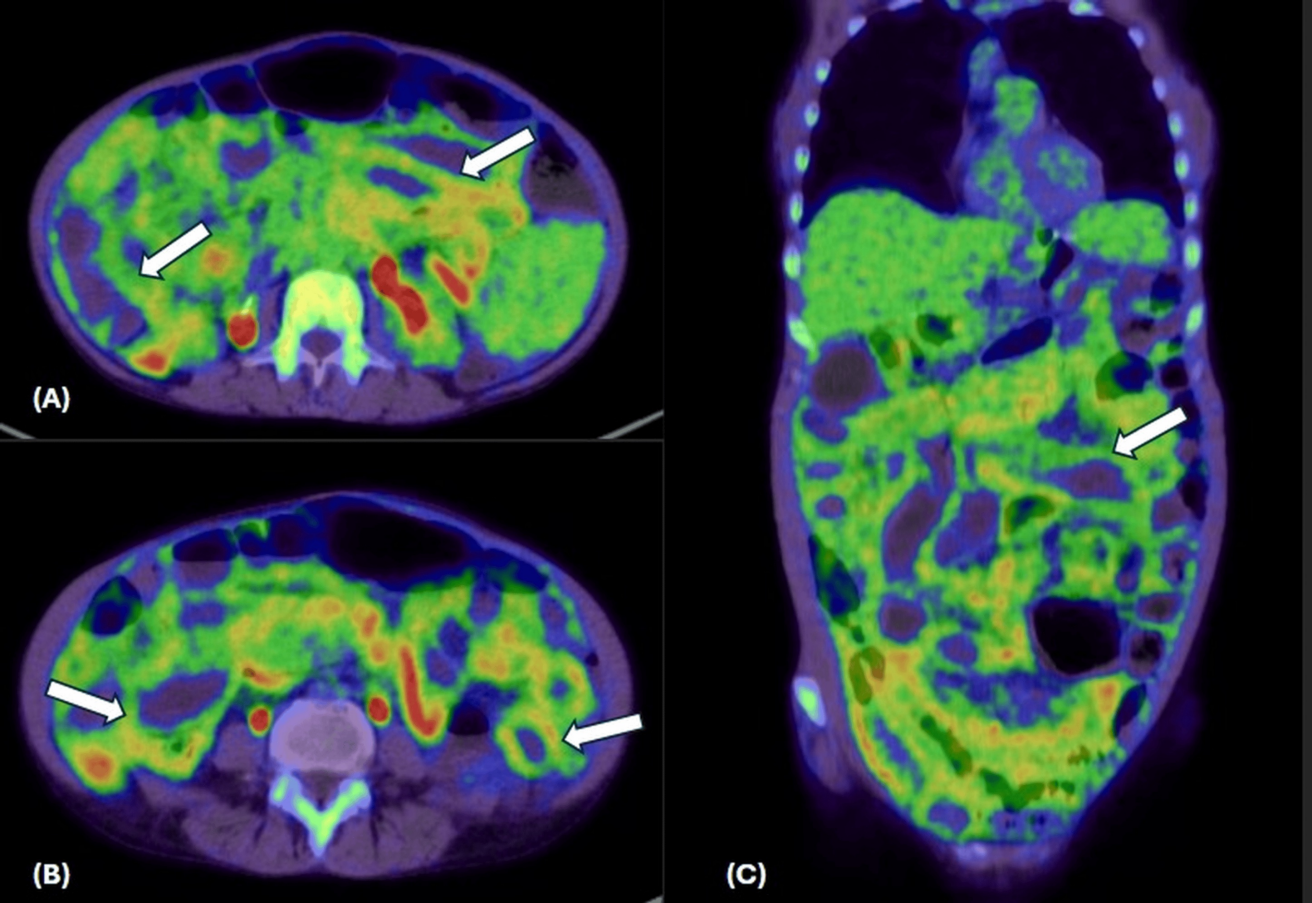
FDG PET/CT scan Axial cuts (A), (B), and coronal cuts of fused PET/CT images showing thickened small bowel with avid FDG uptake (white arrows).
